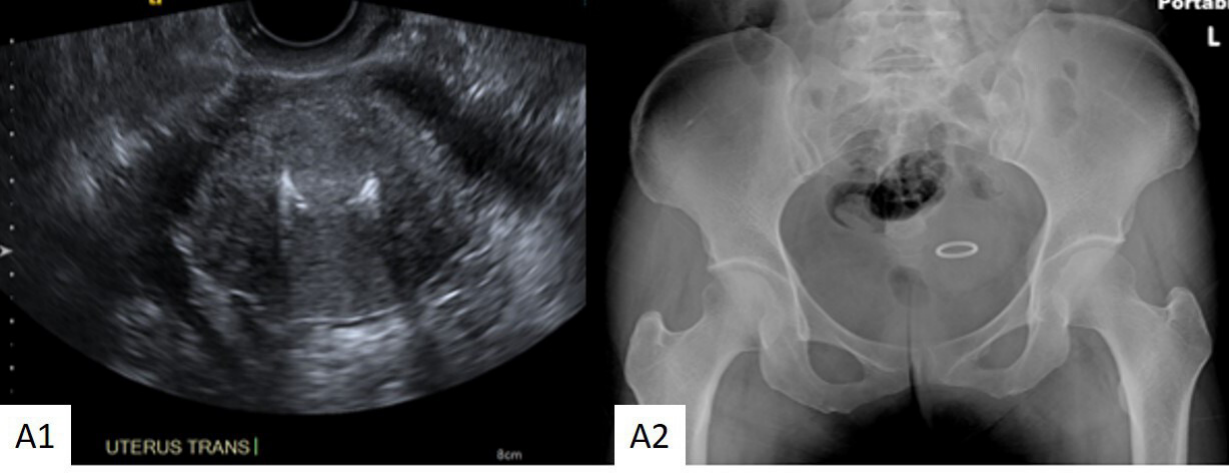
Appearance of Ring IUD 48-year-old female with a ring IUD. A1: Transverse ultrasound image through the uterus demonstrates a ring IUD within the endometrial cavity. A2: Pelvic AP image demonstrates the appearance of the ring IUD in the same patient.
